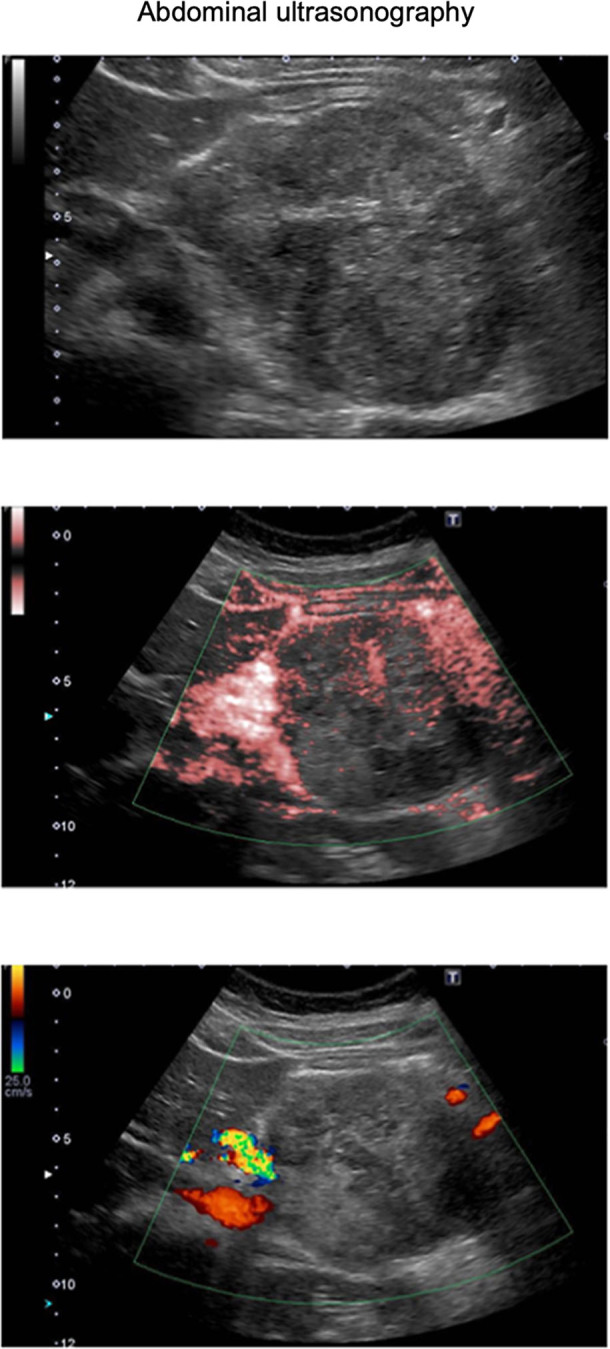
Abdominal ultrasonography: abdominal ultrasonography revealed that the tumor suggested low blood flow signal.
